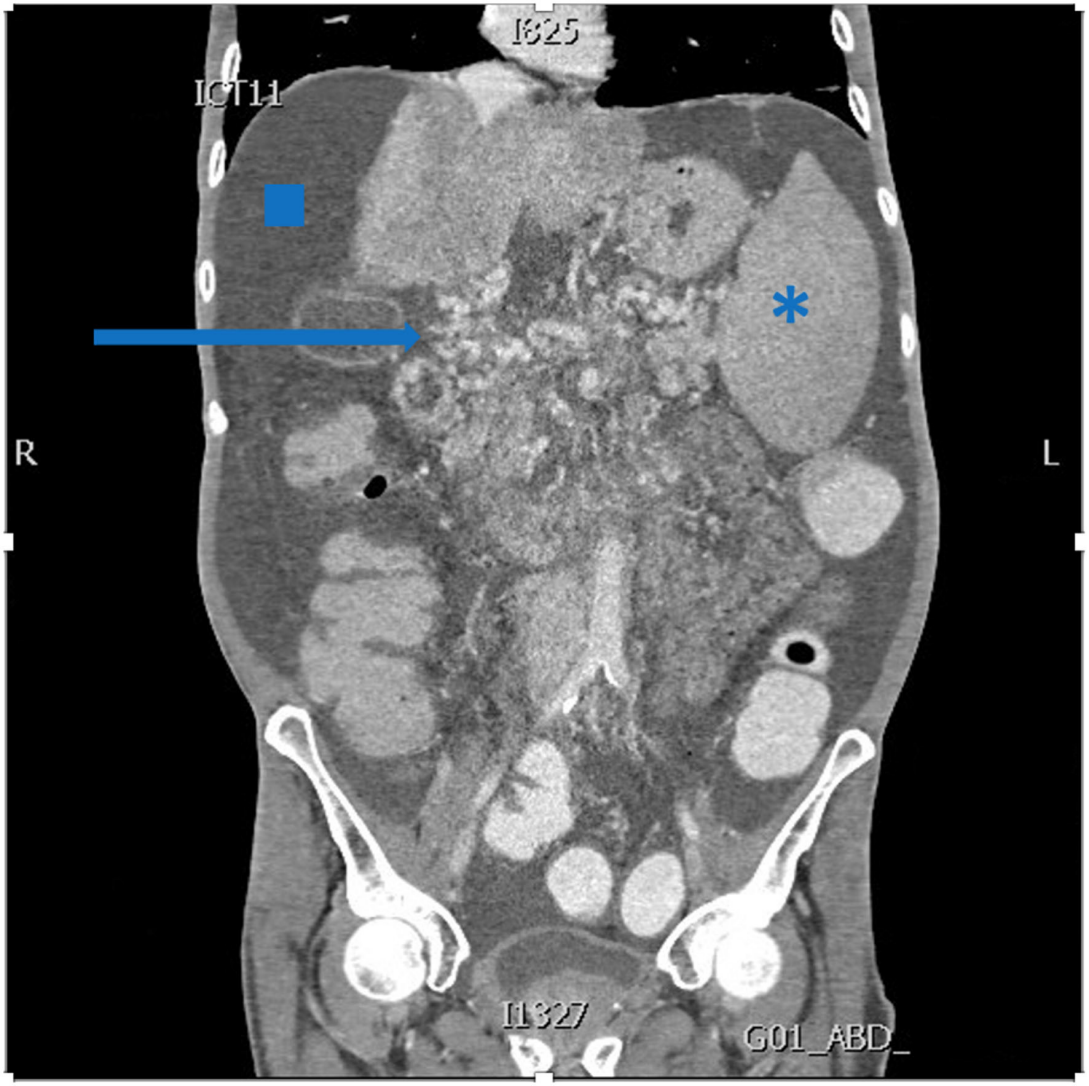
Abdominal computed tomography demonstrating extensive portal collaterals (arrow), ascites (square) and splenomegaly (asterisk).
